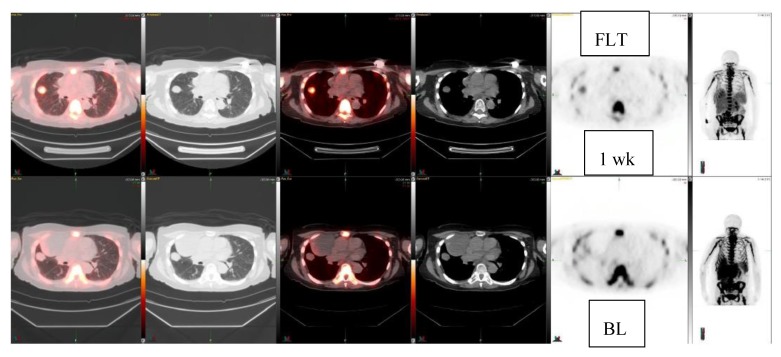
Fibrous tumor, pleura. FLT-study at baseline and at 1 week (2 rows) demonstrates decrease (−43%) in the lung mass: FLT: SUVmax 3.0 ->1.7. On CT it becomes slightly bigger, CT: 2.4 cm × 1.9 cm ->2.5cm × 2.0 cm.
